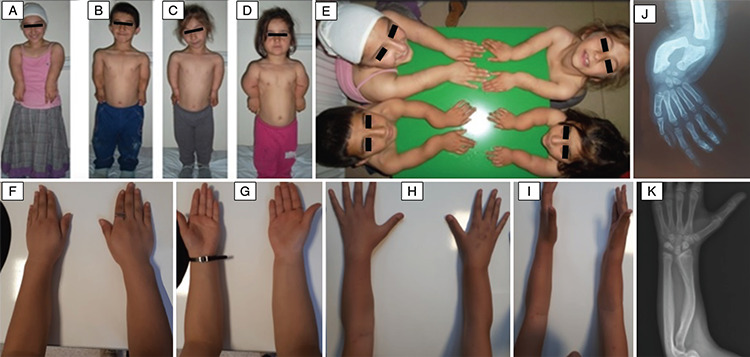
The clinical and radiological findings of the mutation positive patients. A, B, C, D, E) The clinical features of the patients with langer mesomelic dysplasia. F, G) Madelung deformity of patient 15. H, I) Madelung deformity and short forearm of patient 13. J) The direct radiography of patient 2 revealed bowing and shortening of the distal radius, widening of the distal radial-ulnar joint, and triangulation of the distal radial epiphysis, producing an ulnar slant to the articular surface. K) Bowing of forearm, radial bowing and ulnar shaft thickening of patient 13
